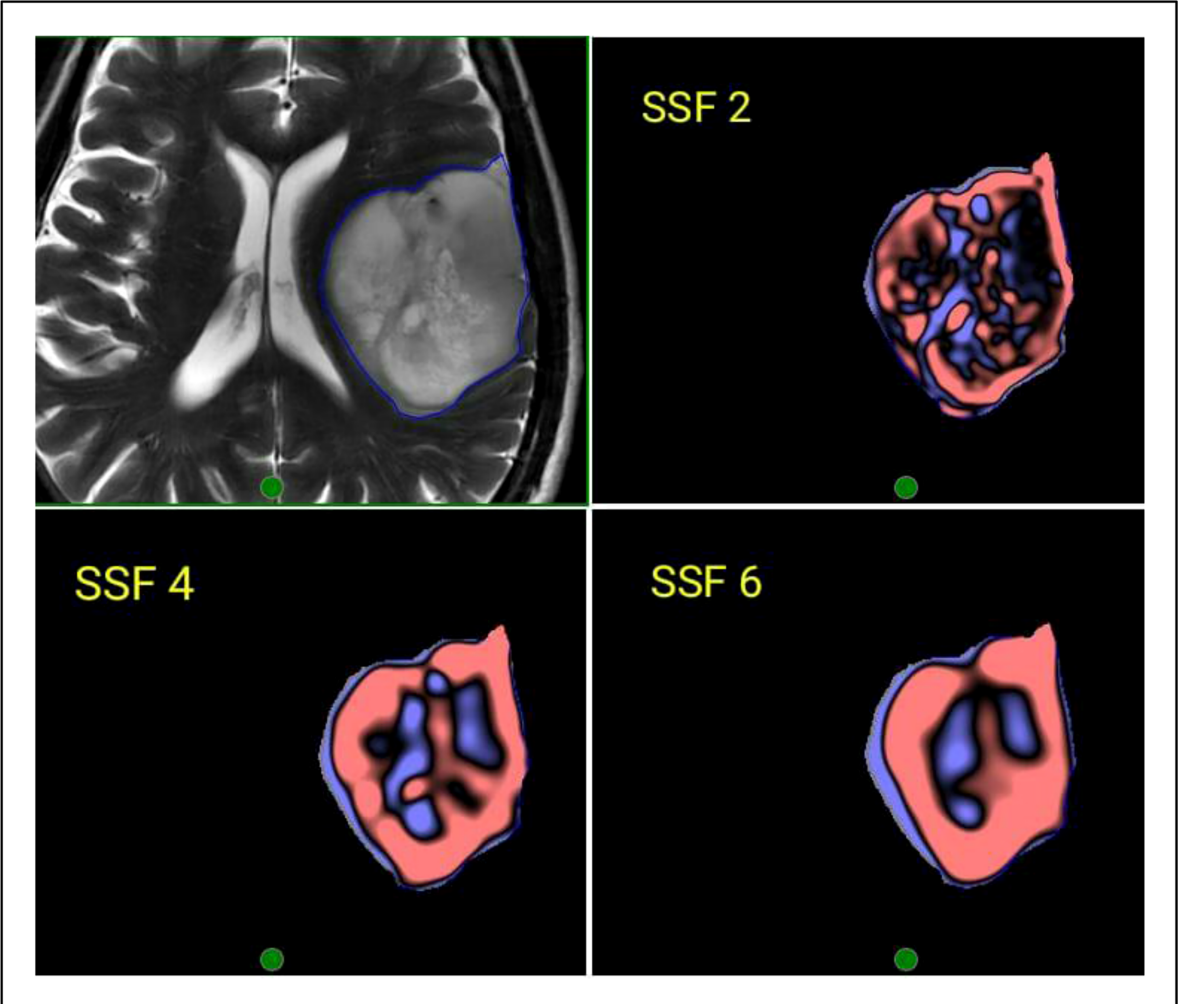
Representative image of the region of interest (ROI) contoured on a T2W MRI and corresponding filtered images using Laplacian of Gaussian (LOG) bandpass filtration algorithm showing SSF-2 (fine texture), SSF 4 (Medium texture), and SSF 6 (Coarse texture).
